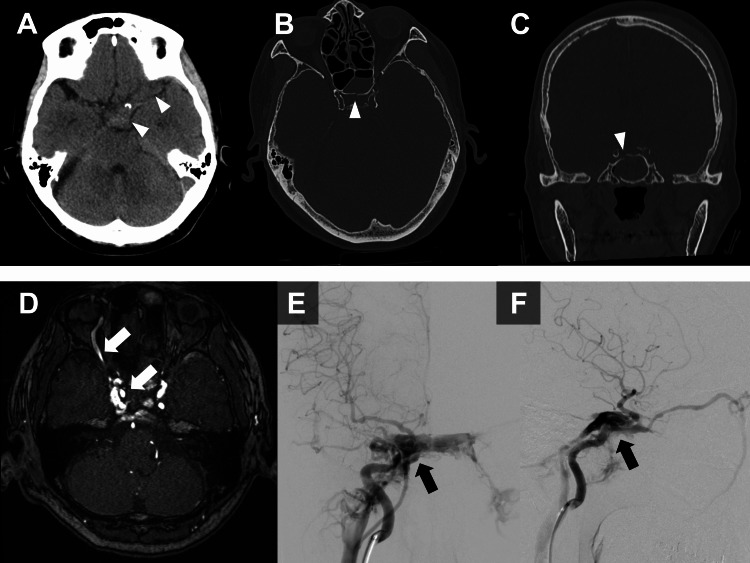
Initial imaging of a traumatic direct carotid-cavernous fistula. (A) Axial unenhanced computed tomography (CT) showing subtle hyperdensity in the suprasellar cistern (arrowheads), consistent with traumatic subarachnoid hemorrhage. (B) Axial CT (bone window) demonstrating a sphenoid bone fracture (arrowhead). (C) Coronal CT (bone window) demonstrating a sphenoid bone fracture (arrowhead). (D) Axial magnetic resonance angiography (MRA) source image on day 9 of hospitalization showing an arteriovenous shunt in the cavernous sinus region with a dilated right superior ophthalmic vein (white arrows). (E) Anteroposterior right internal carotid artery (ICA) angiography confirming a direct carotid-cavernous fistula between the cavernous ICA and right cavernous sinus (black arrow). (F) Lateral right ICA angiography confirming a direct carotid-cavernous fistula (black arrow).
